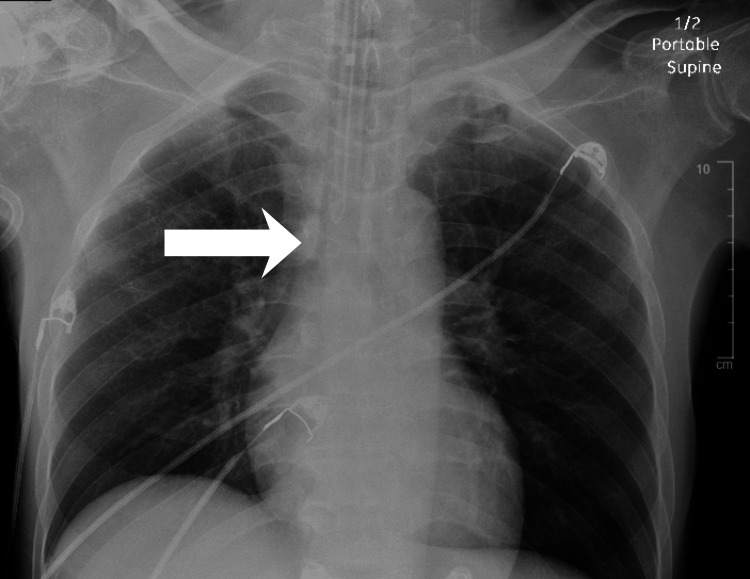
Portable supine chest radiograph on admission demonstrating appropriate endotracheal tube positioning and clear lung fields. The arrow highlights the distal endotracheal tube tip, which is positioned appropriately above the carina. Both lung fields appear clear without evidence of pneumothorax, consolidation, or pulmonary edema, supporting a non-pulmonary cause of the patient’s respiratory failure and altered mental status.
